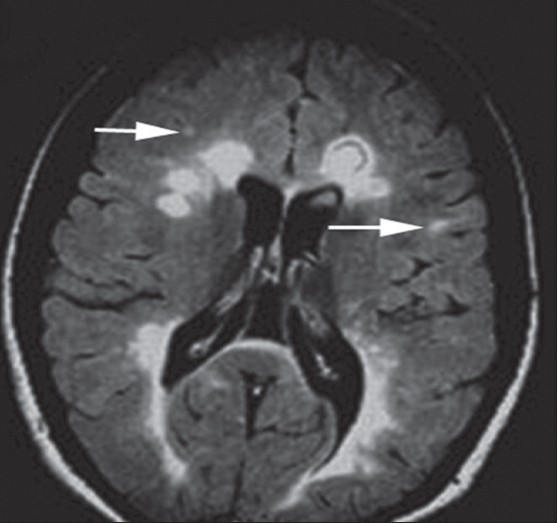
FLAIR MR image showing bright signal from the plaques of MS (white arrows)
